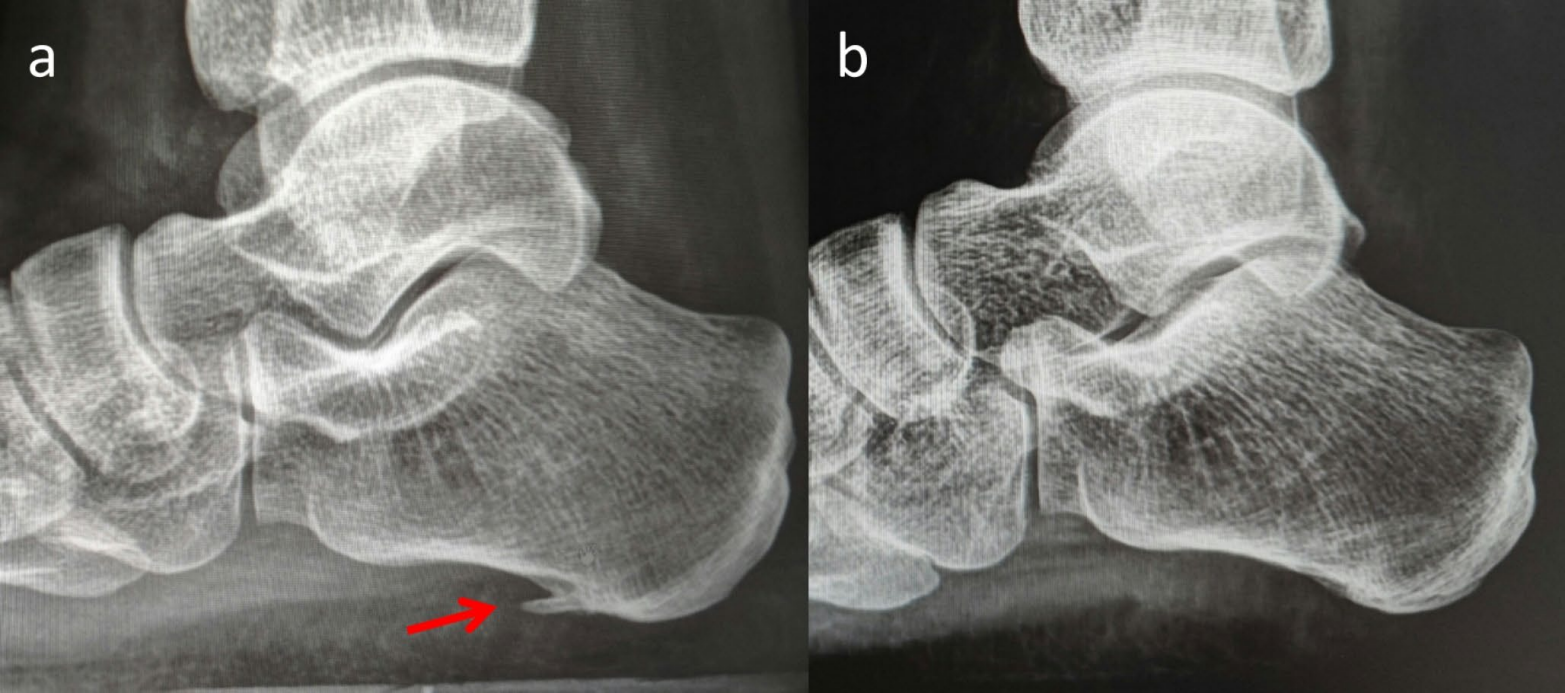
X-ray comparison before and after Calcaneal spur removal. (a) Preoperative X-ray shows a prominent bone spur beneath the calcaneus (indicated by the red arrow). (b) Postoperative X-ray shows that the bone spur has been removed, the plantar bony prominence has disappeared, and the bony structure appears smoother compared to the preoperative state
